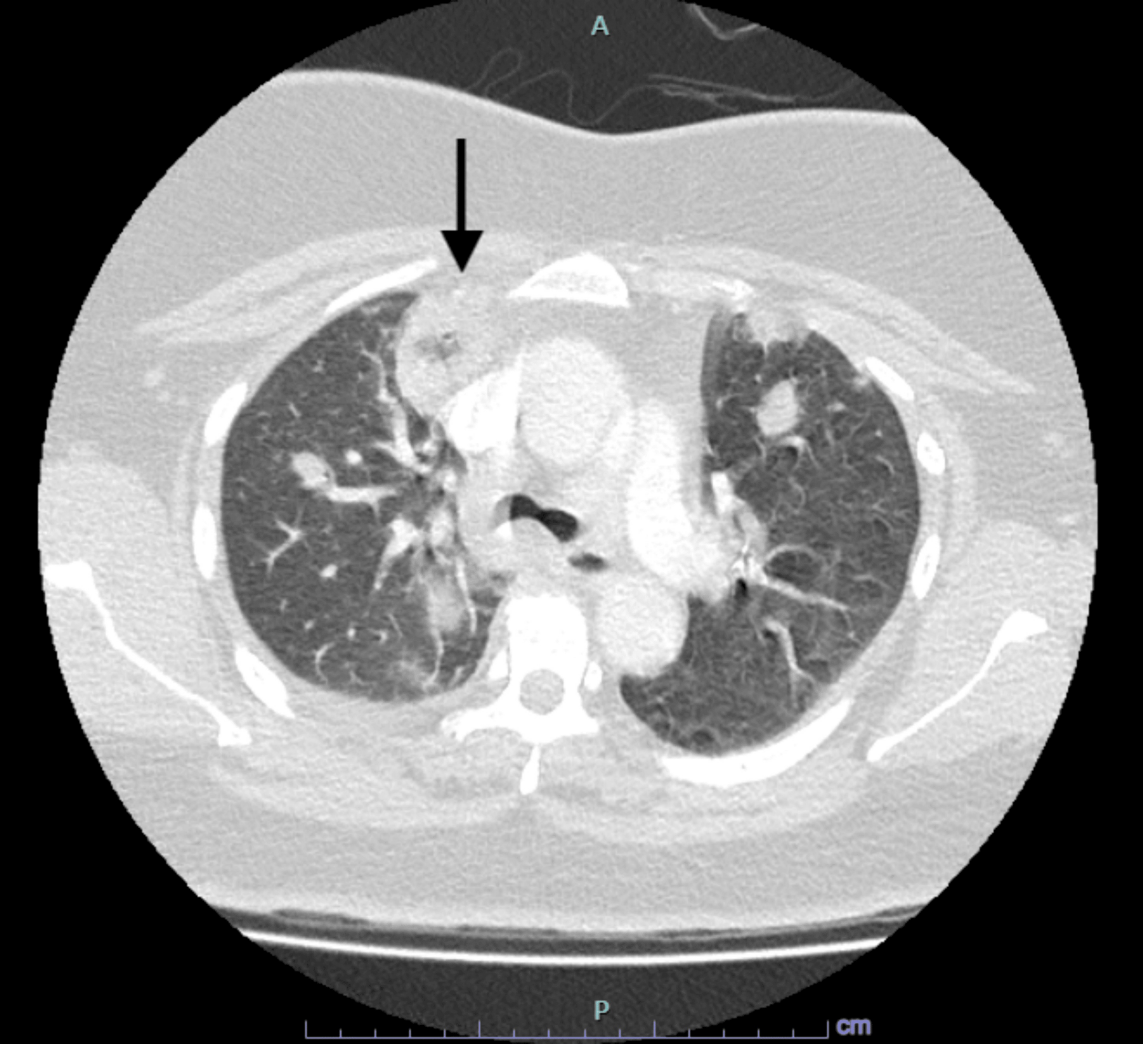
Initial CT scan of thorax showing bilateral cavitary pneumonia with predominant nodule at the right upper lobe, measuring 3.5 x 2.7 x 2.3 cm. A: anterior, P: posterior.
